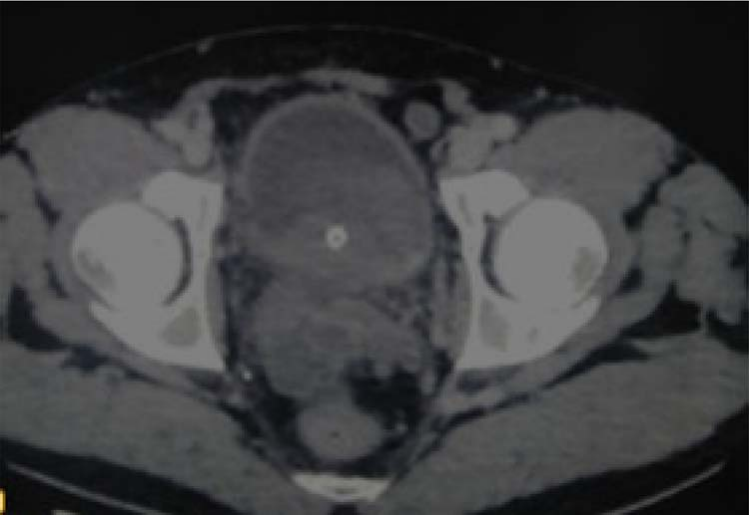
CT revealing a bladder tumor of 6 × 5 cm over the trigone and left wall.
